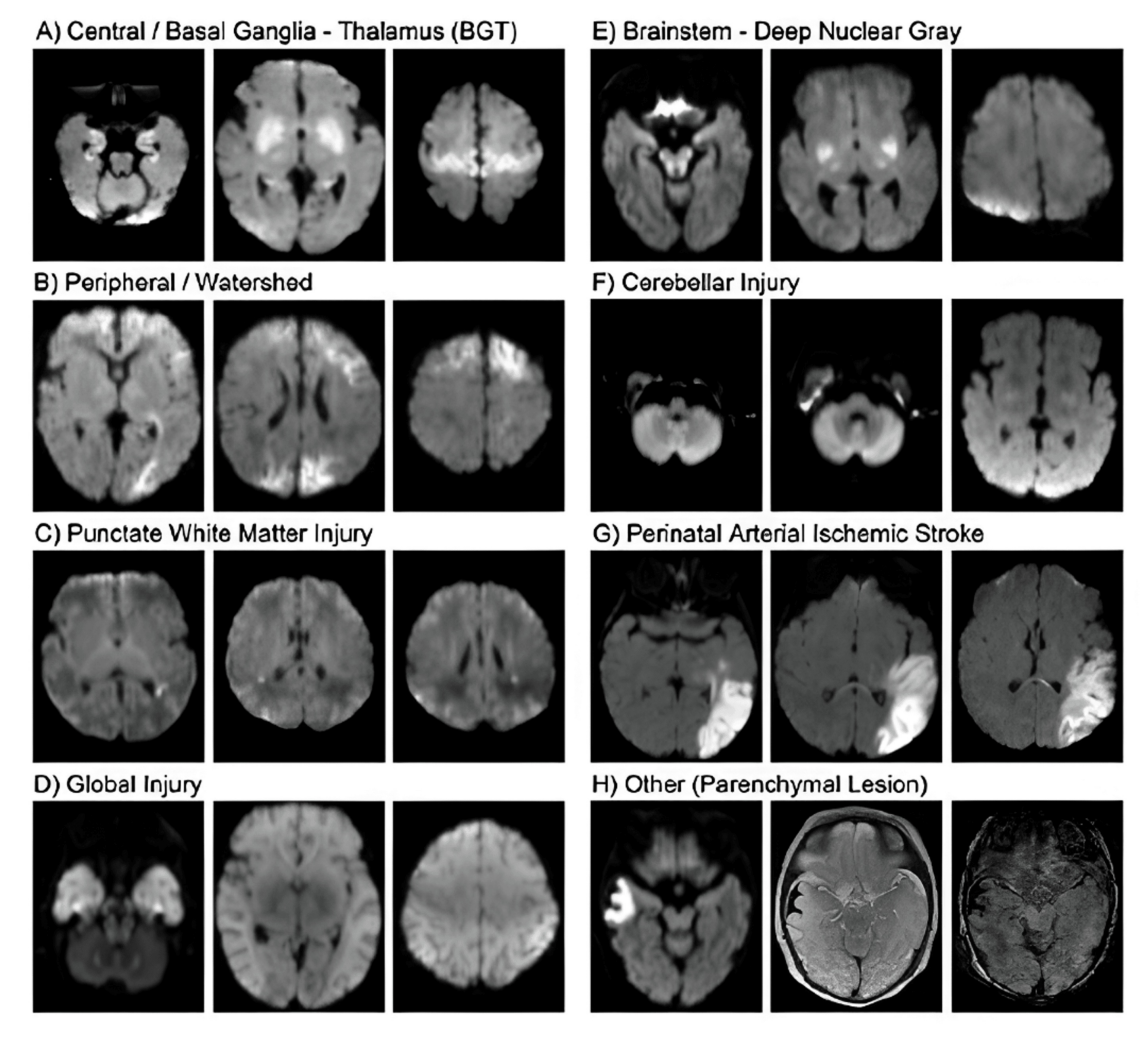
Patterns of brain injury in neonates on MRI DWI sequences (a) Central/basal ganglia-thalamus (BGT); (b) Peripheral/watershed; (c) Punctate white matter injury; (d) Global injury; (e) Brainstem-deep nuclear gray; (f) Cerebellar injury; (g) Perinatal ischemic stroke; (h) Other (parenchymal lesion)—subpial hemorrhage. DWI: diffusion-weighted imaging Reproduced from Seminars in Fetal and Neonatal Medicine, Volume 26, Issue 5, Wisnowski, JL et al., Neuroimaging in the term newborn with neonatal encephalopathy, 101304, Copyright (2021), with permission from Elsevier [3].
